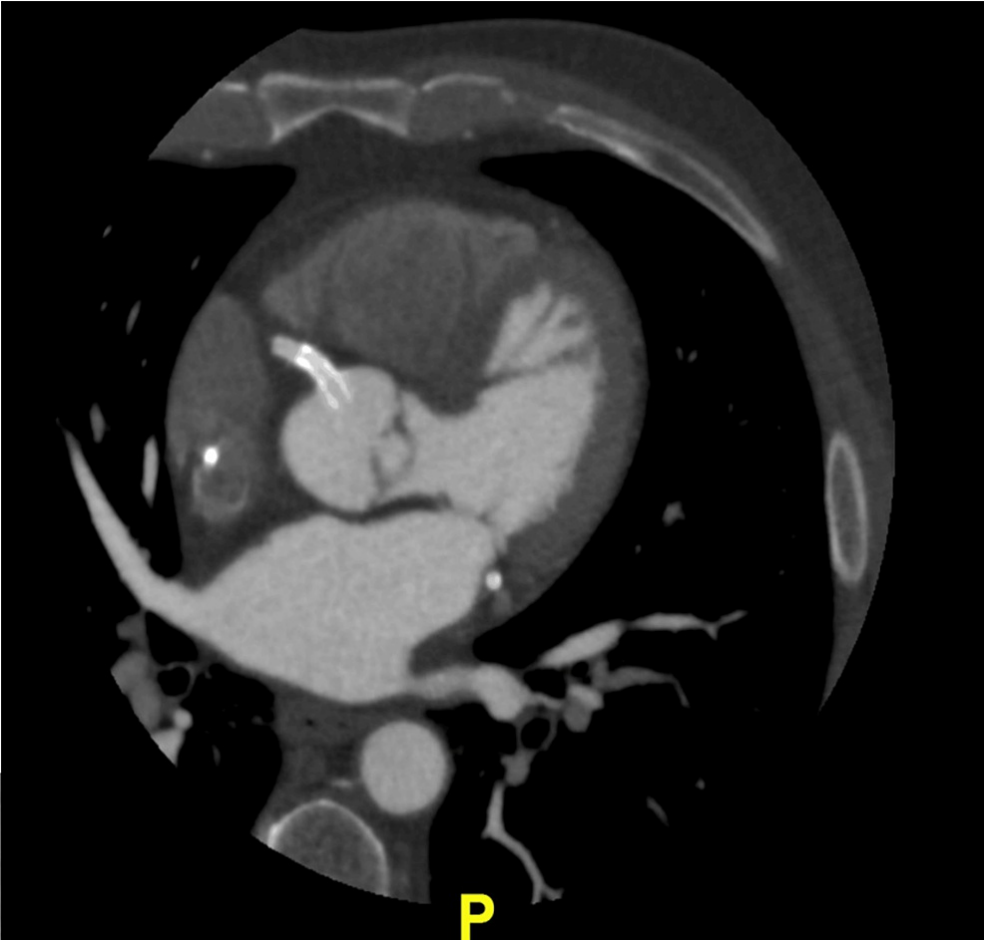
Coronary computed tomographic angiography of coronary arteries in the axial view Coronary computed tomographic angiography (CCTA) of coronary arteries in the axial view showing an echodense, tubular structure protruding from right coronary artery (RCA) into right coronary sinus and then into the aortic lumen. Based on this imaging, the stent otherwise looks patent and well-opposed to the RCA wall.
